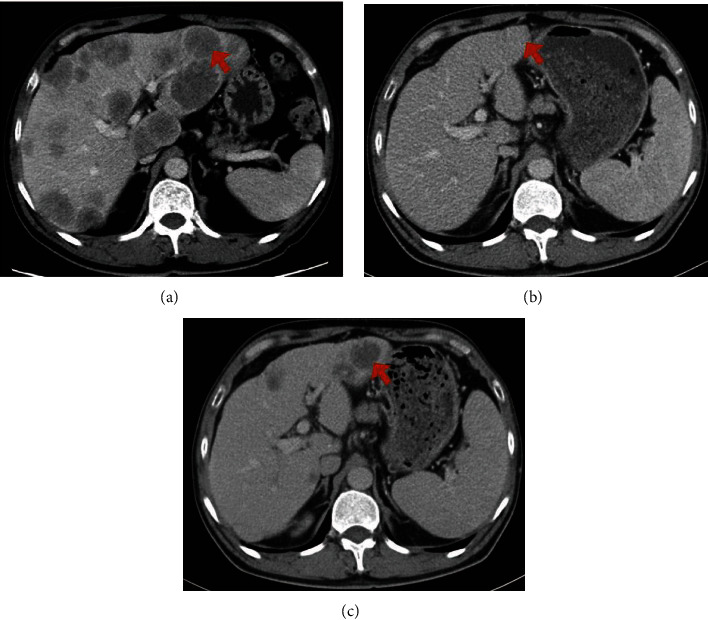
Examples of DLM on preoperative image in patients with CRLM. (a) Image showing the CRLM in the first diagnosis time (arrow). (b) Image showing DLM after conversion therapy (arrow). (c) Image showing recurrence of DLM with follow-up (arrow). Abbreviations: CRLM: colorectal liver metastases; DLM: disappearing liver metastasis; MRI: magnetic resonance imaging.
